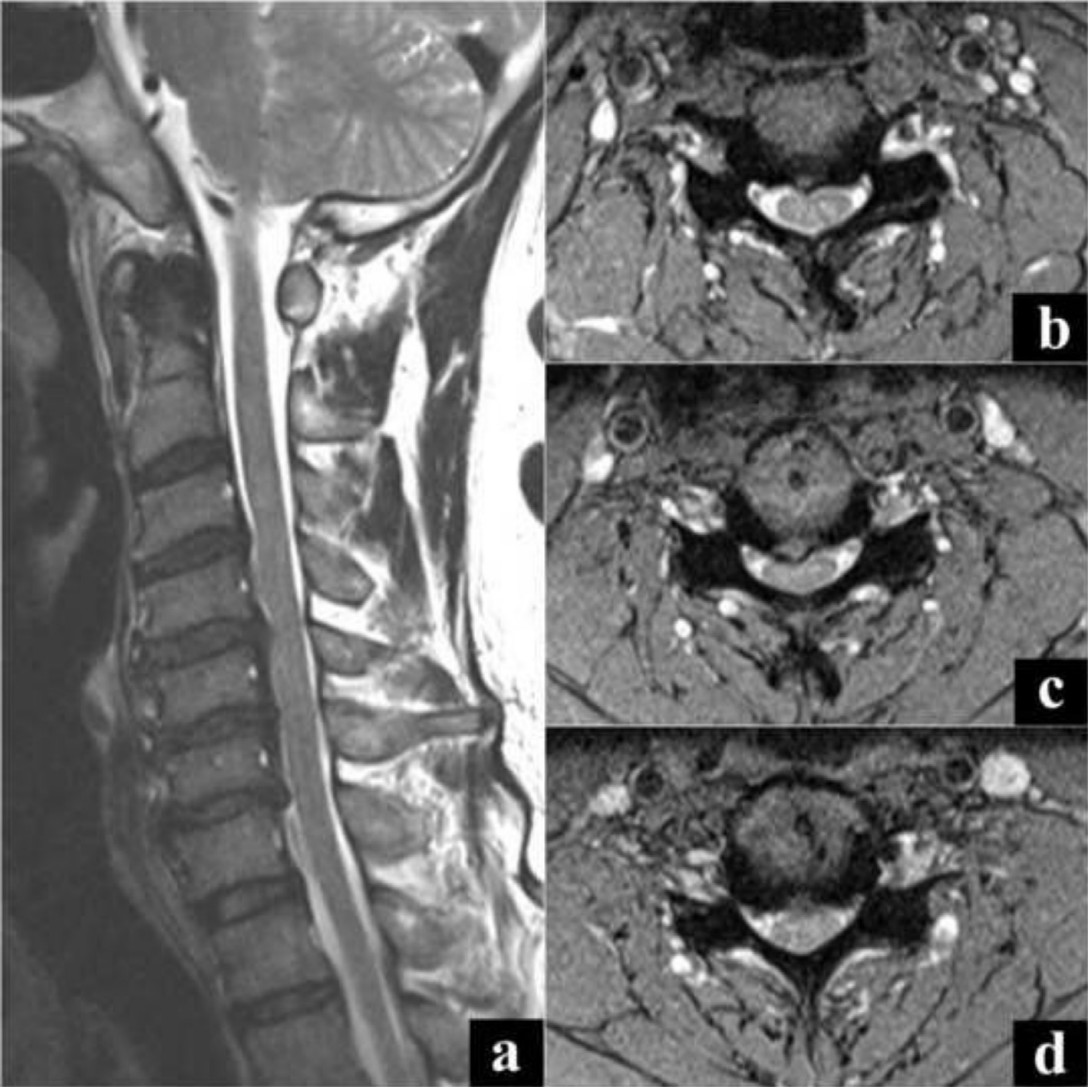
MR image showing mild spinal canal stenosis at C3–C6 levels. a) Sagittal cut image, b) Axial cut at C3–C4 level, c) Axial cut at C4–C5 level, d) Axial cut at C5–C6 level.
